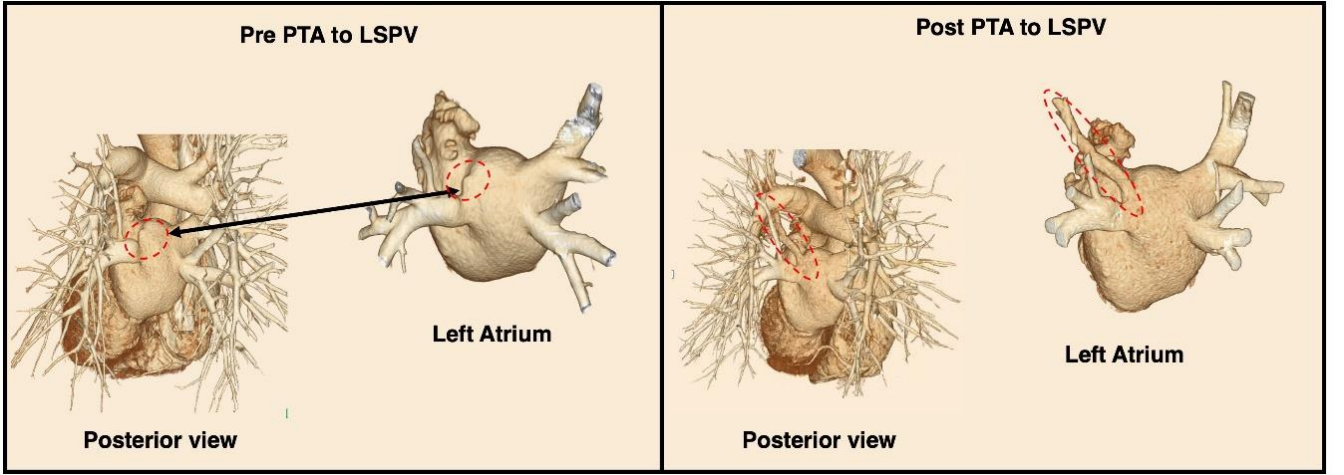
Case 1. 3-D Rendering of Pre- and Post-PTA CT Scan Images
